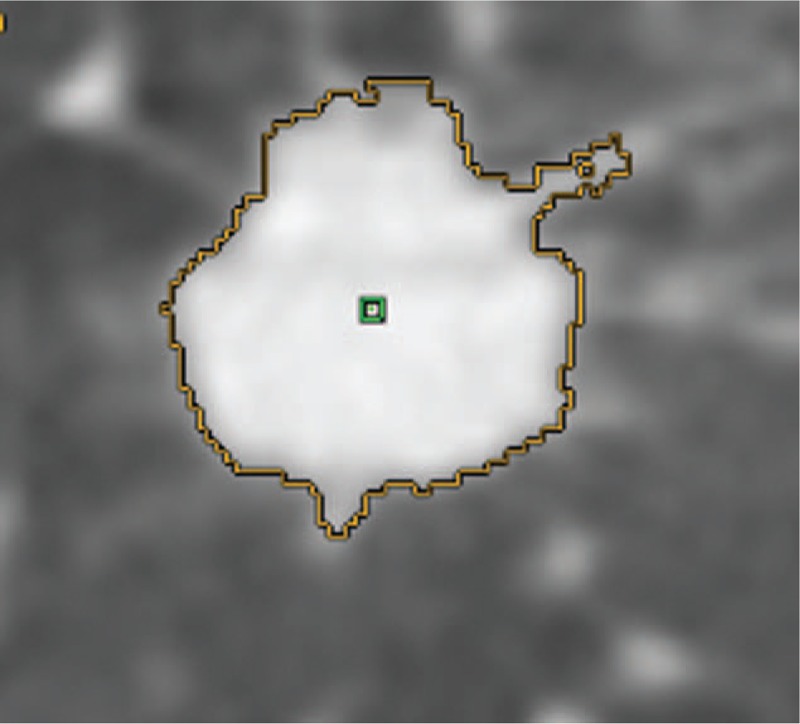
The lesion computed tomography volume (CTV) was measured through Lung VCAR software. CTV = computed tomography volume, VCAR = Lung volume computerized assisted reporting.
